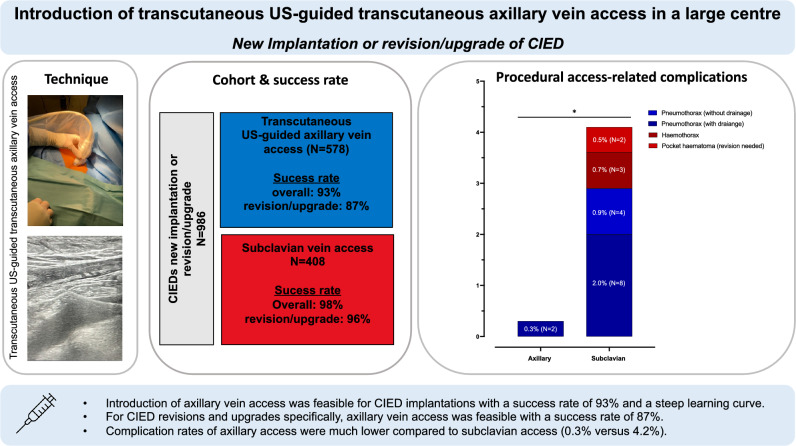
CIED* cardiac implantable electronic device, * US* ultrasound
